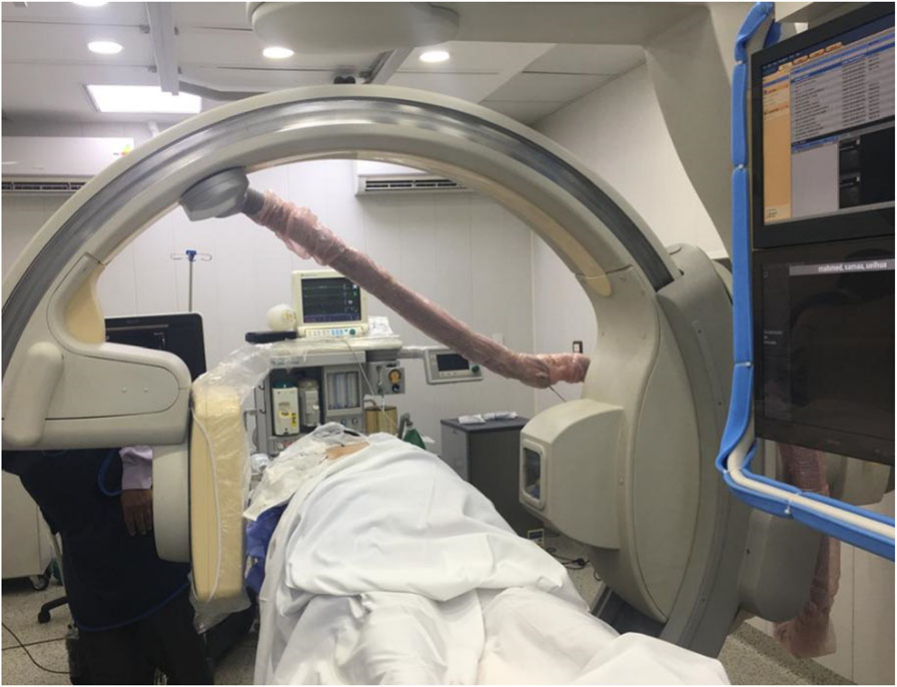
Allura Xper FD 20 Flat detector Fluoroscopy with Xper CT, Philips, Netherlands
